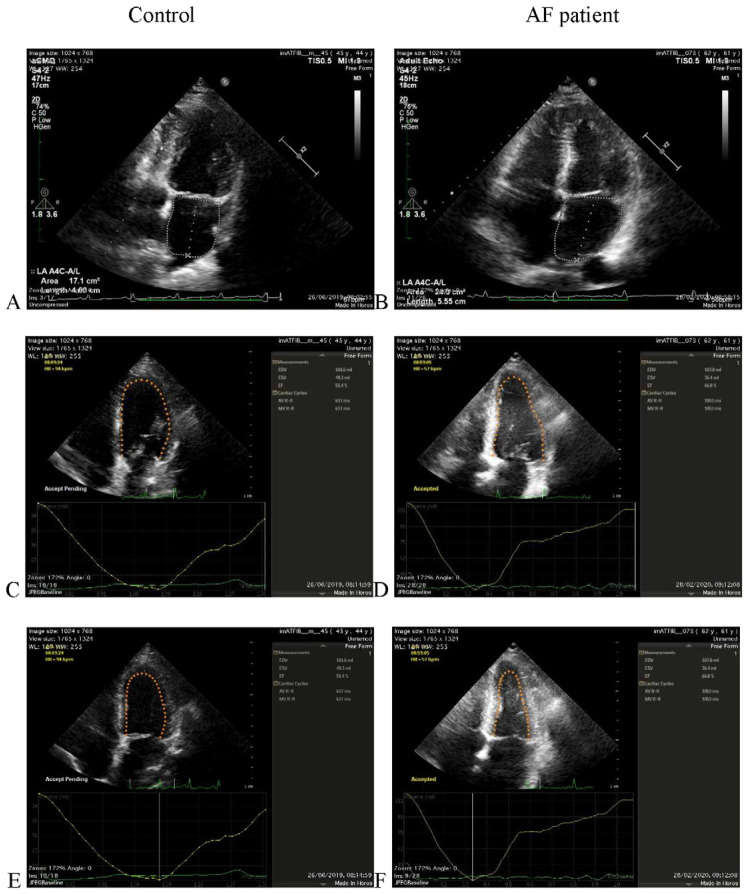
Representative echocardiography images from a healthy subject (Control—left panel) and from an AF patient (right panel) used to determine LA Vol (A,B), EDV (C,D), and ESV (E,F), respectively.
